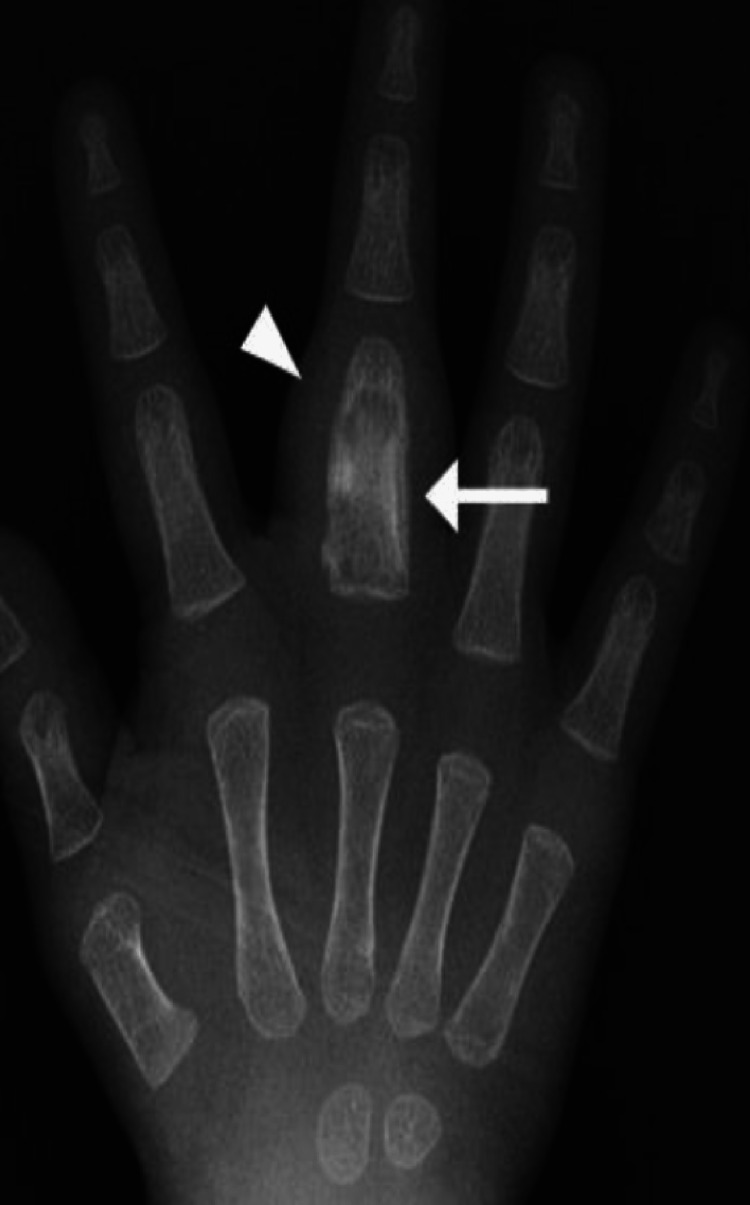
A 17-month-old girl diagnosed with BCG osteomyelitis of the phalanx The radiograph of the right hand shows destructive change and fusiform expansion of the proximal phalanx of the middle finger, which is depicted as multiple lytic lesions in the marrow and diaphyseal expansion (arrow). Noted is a single layer periosteal reaction (arrowhead) and prominent soft tissue swelling [17].
